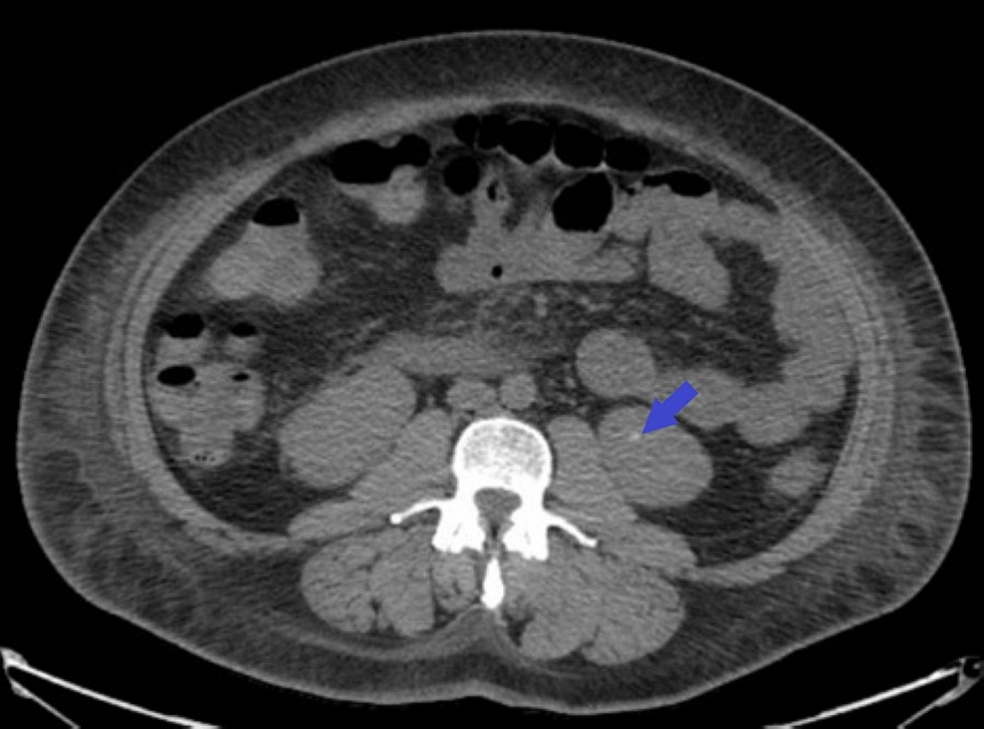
CT abdomen showing punctate left renal lower pole calcification (blue arrow).
